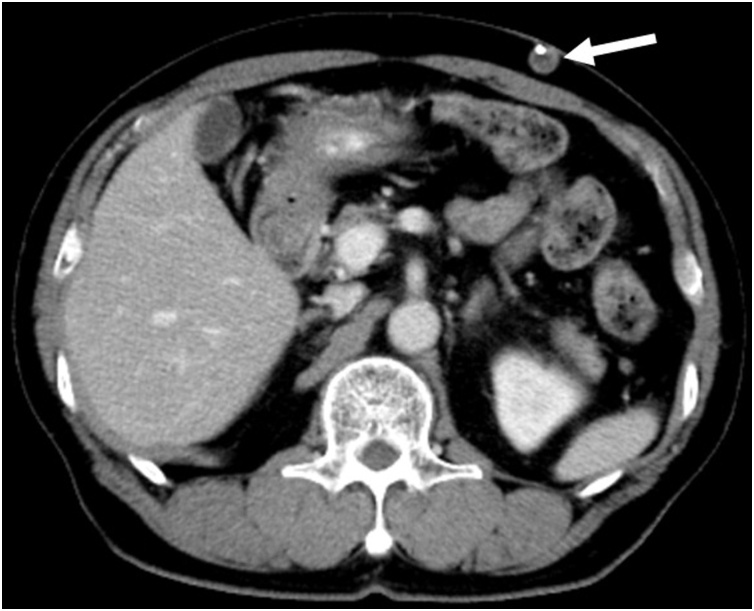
Computed tomography shows a mass in the subcutaneous tissue of the abdominal wall along the VP shunt.
